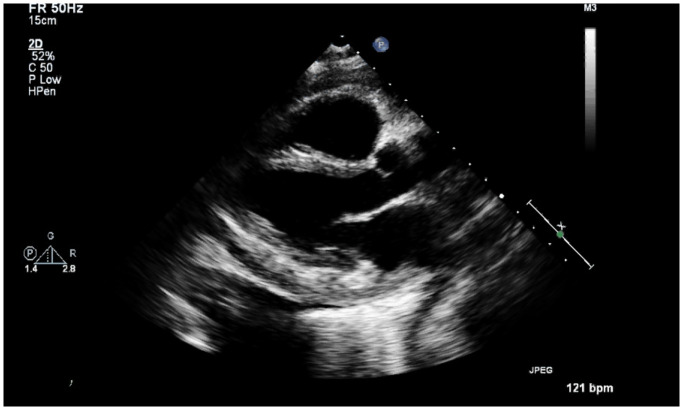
Echocardiography shows markedly thickened pericardium and abnormal soft tissue echoes anterior mediastinum.
